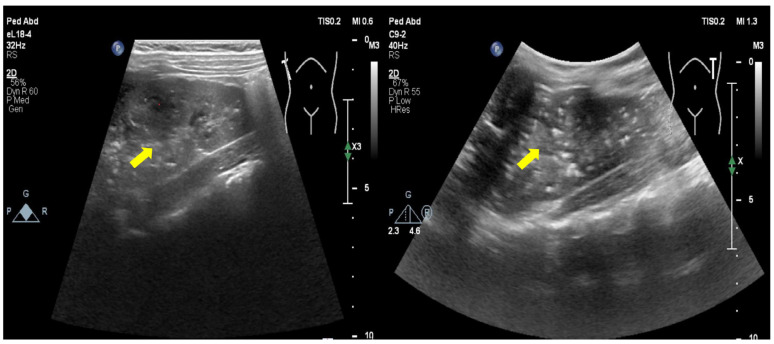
Ultrasound showed scattered calcifications in both kidneys.
